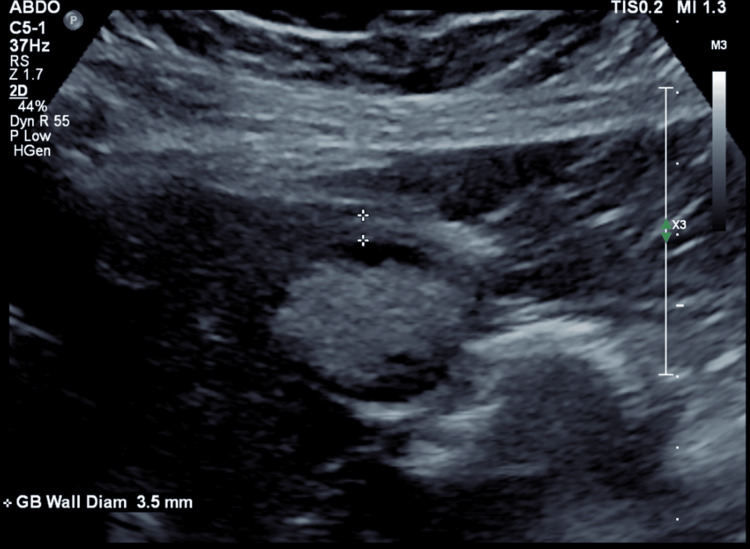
Ultrasound shows gallbladder wall thickness 3.5 mm, suggesting no signs of acute inflammation.
